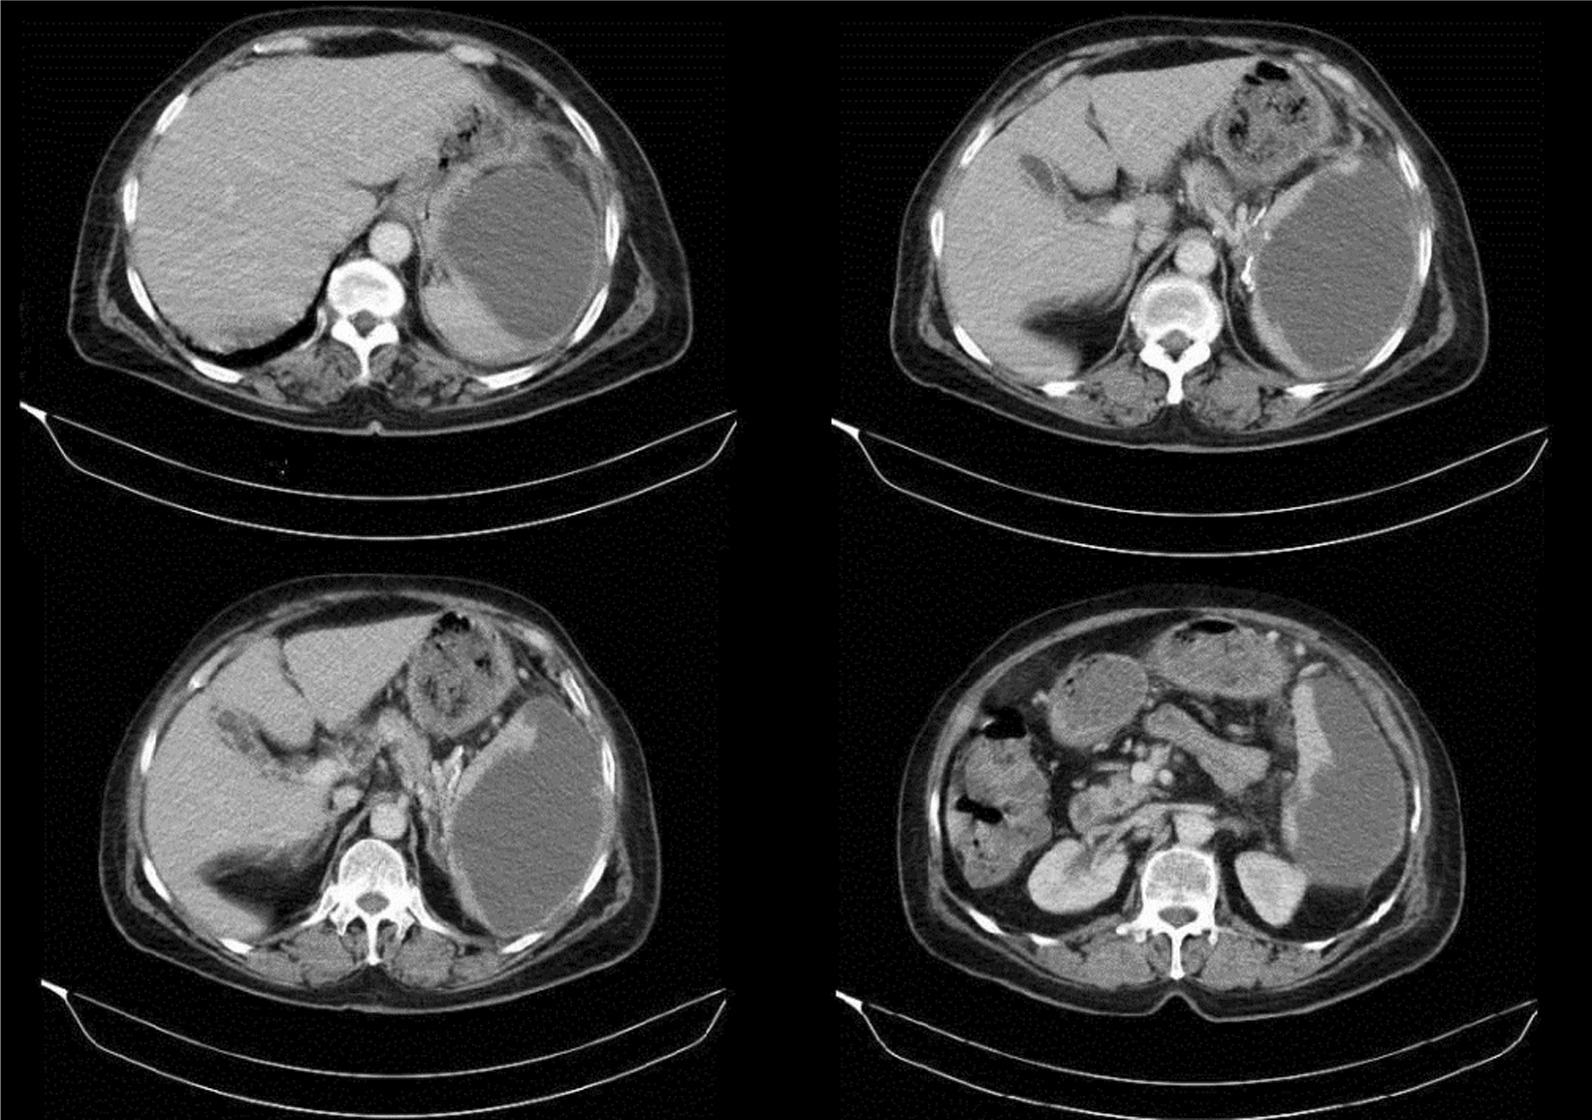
CT (computed tomography) scan showing a large splenic abscess measuring 10.8 × 8.7 × 5.3 cm
